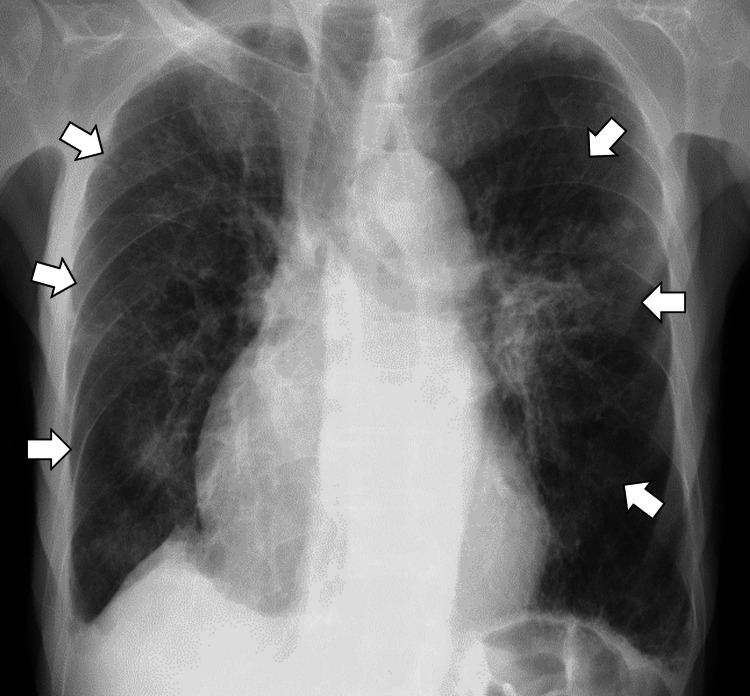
Follow-up chest radiography demonstrating significant resolution of the pulmonary infiltrates by hospital day 7
